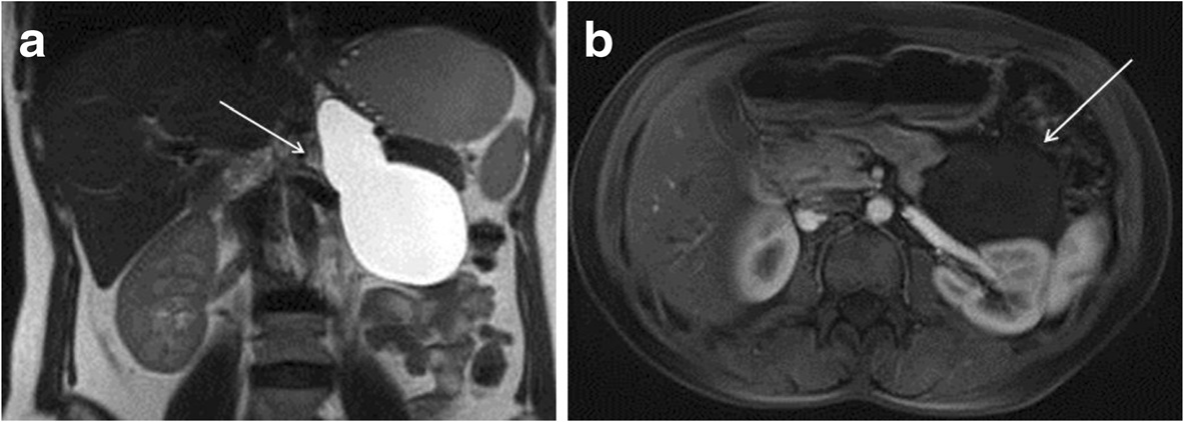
Magnetic resonance (MR) imaging of the cystic lymphangioma. Coronal (a) T2-weighted turbo spin-echo and axial (b) T1-weighted contrast-enhanced gradient-echo MR images show an ovoid left adrenal lesion (arrow). It is homogeneously hyperintense on T2-weightening (a) and hypointense on T1-weightening (b), thus confirming the fluid content without loculation neither solid component. The thin wall surrounding the lesion is barely perceptible.
